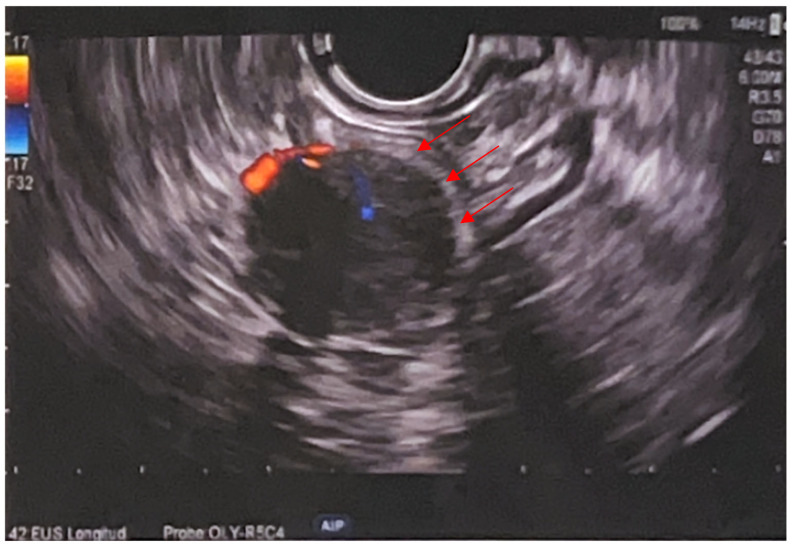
Endoscopic ultrasound (EUS) showing a well-defined, hypoechoic, vascularized submucosal lesion (20 × 16 mm) arising from the muscularis propria of the sigmoid colon (arrows).
